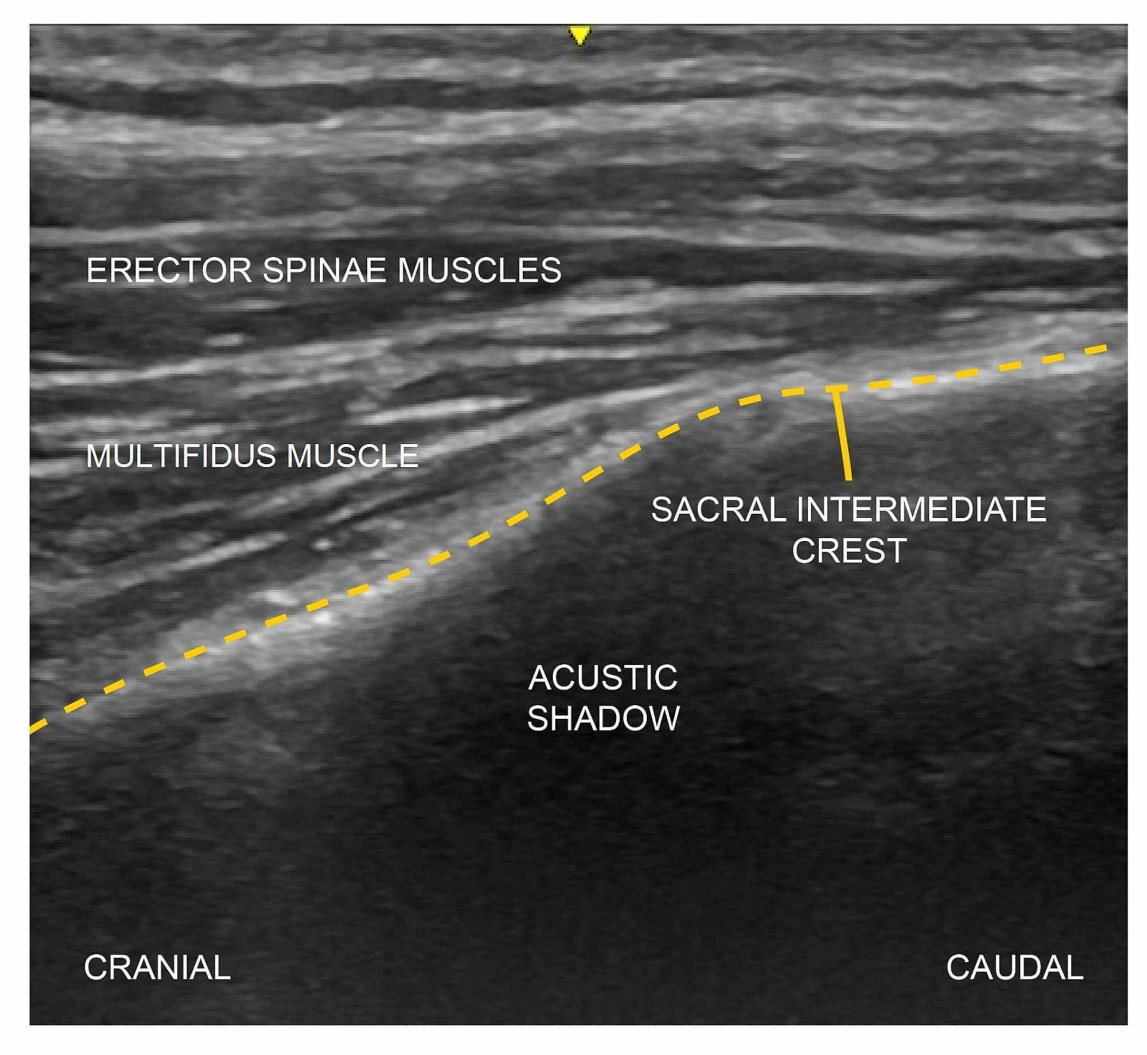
The sonoanatomy relevant for the ultrasound-guided sacral erector spinae plane block (ESPB). Dashed lines represent the sacral intermediate crest.
